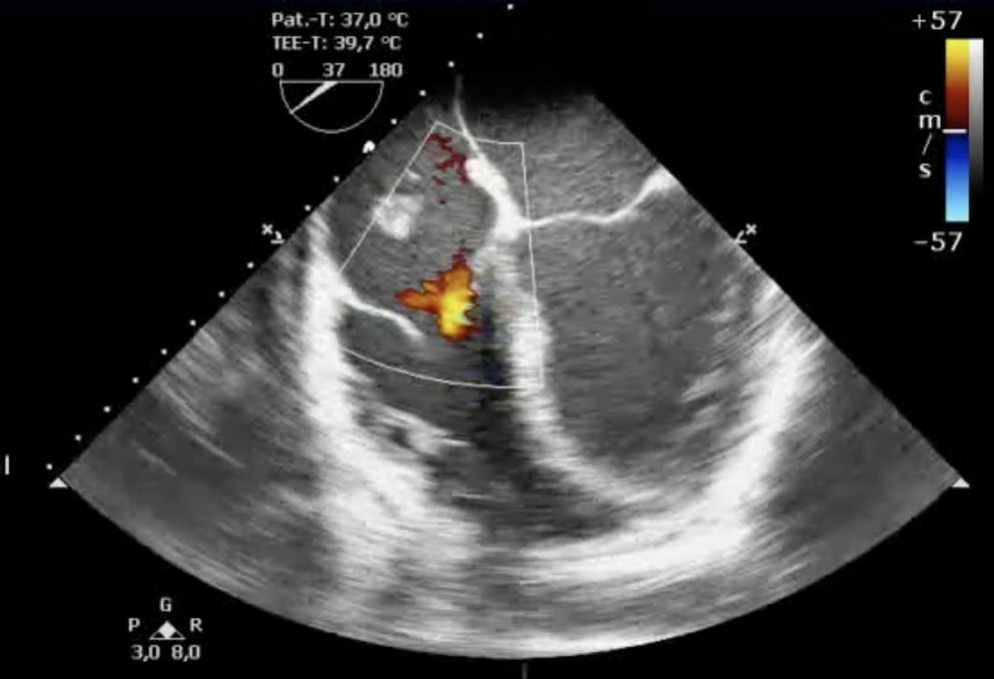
Transesophageal echocardiography: midesophageal four‐chamber view with no relevant regurgitation of the tricuspid valve. Color Doppler of the tricuspid valve in the midesophageal four‐chamber view revealed no relevant regurgitation. The tubular structure was partially visible in the right atrium. An additional movie file is included, displaying the integrity of the tricuspid valve assessed by color Doppler (see Video S2)
